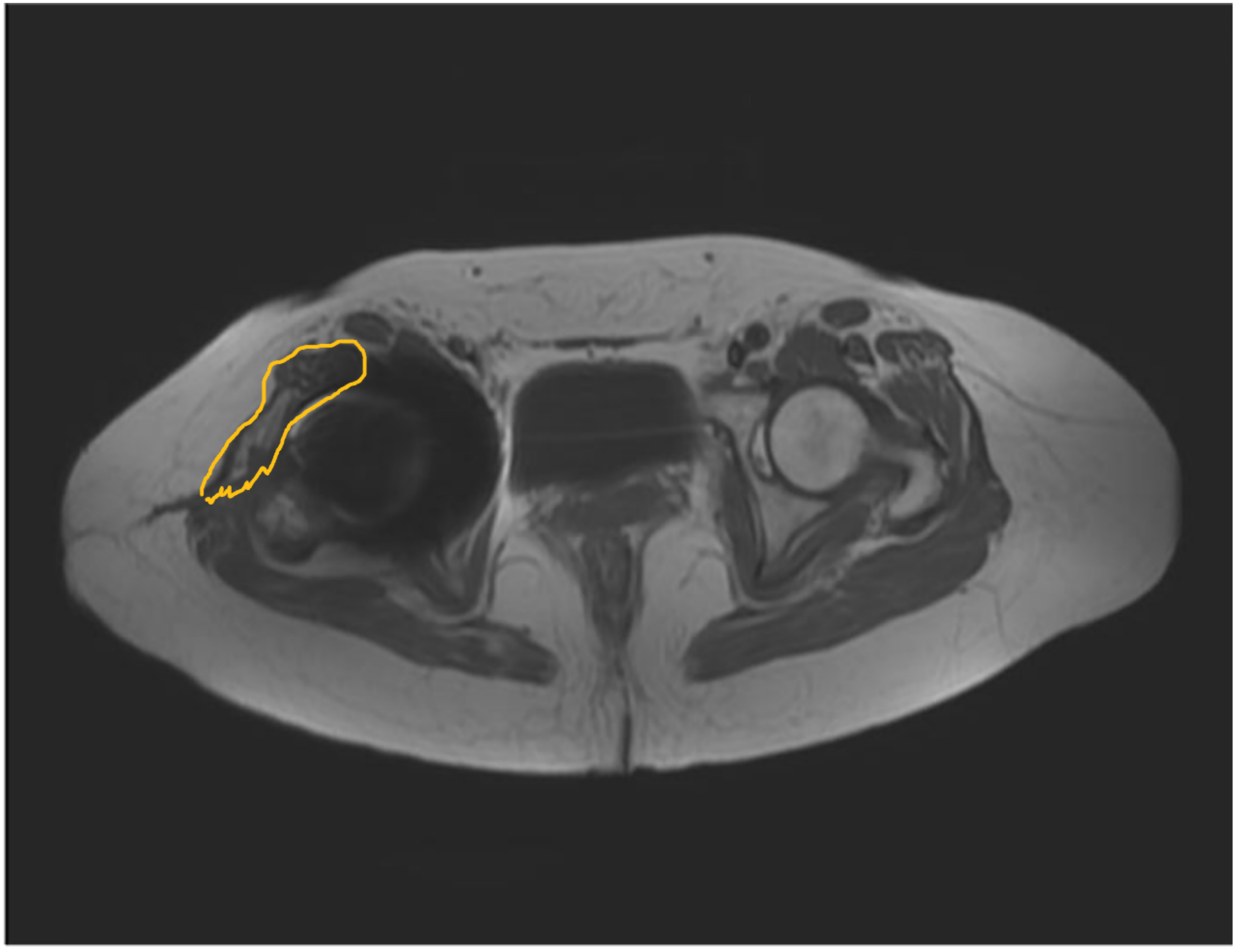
Continuity of the musculotendinous flap (yellow line) was evaluated by magnetic resonance imaging (MRI) at 9-month interval postoperatively.
